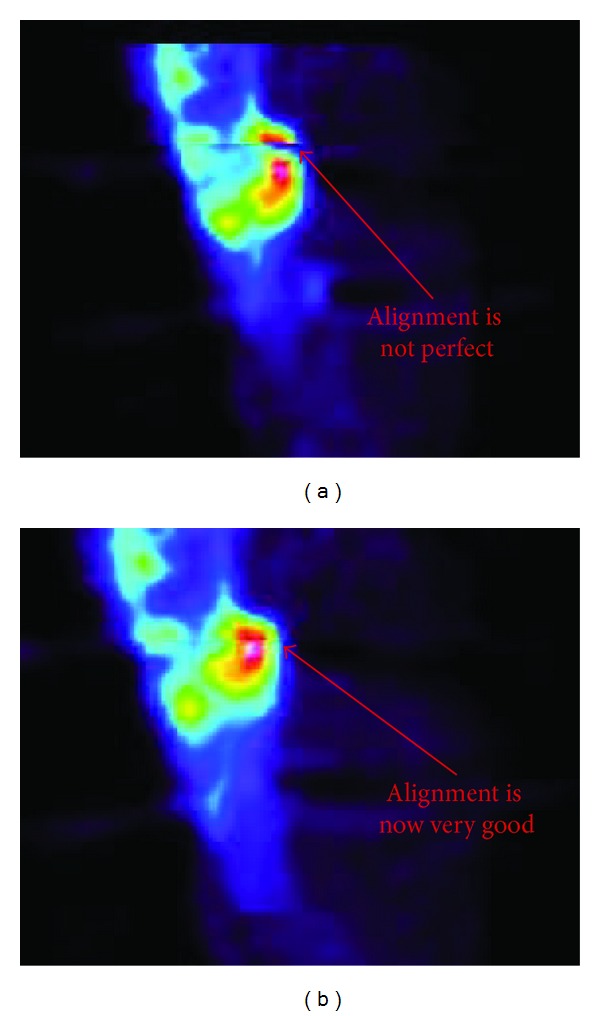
A sagittal section of the first PET scan for Patient 1 is superimposed on the second one. The first alignment between the exam at 40 days and at 84 days is shown in (a) and the final alignment after a slight manual adjustment in (b). This allows the same VOI to be used for the SUV calculations.
